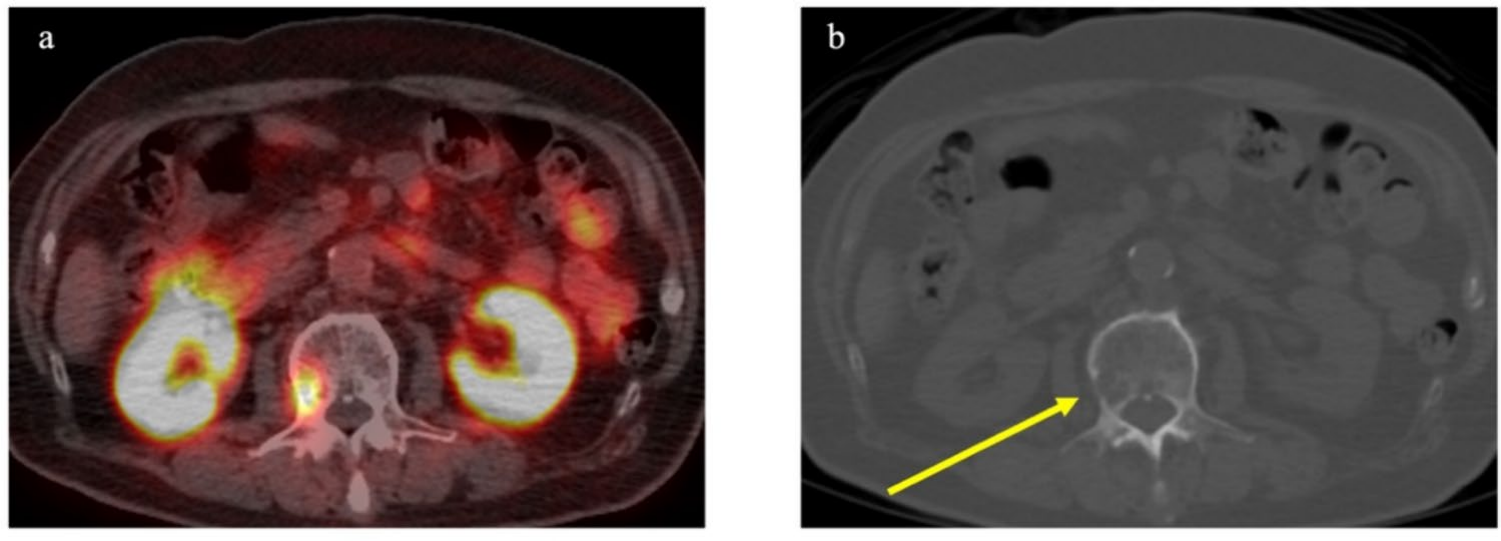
An 80-year-old man who had previously been treated with radiation therapy (cT3bN1, Gleason score 4 + 5) demonstrated biochemical recurrence. PSA level elevated to 4.46 ng/ml. PET/CT using ^18^F-FSU-880 showed focal uptake in a primarily osteolytic lesion of the lumbar spine (SUVmax: 9.4). a Transaxial PET/CT fusion image. b Transaxial CT
